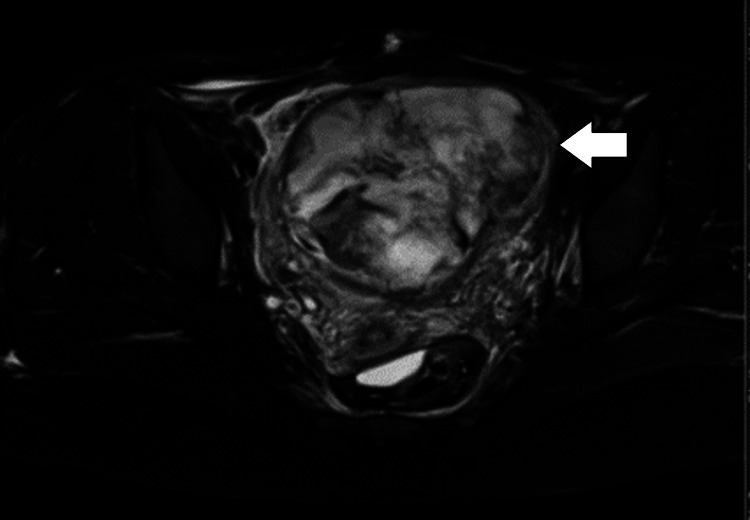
MRI of the pelvis showing previously noted large bladder tumor again seen arising from the left bladder wall. Normal enhancement of the bladder wall was seen with no convincing evidence to suggest an extramural invasion. Adjacent pelvic viscera appears to be displaced and invaded by this large bladder lesion. No large pelvic lymph nodes or destructive bony lesions were seen. MRI, magnetic resonance imaging
